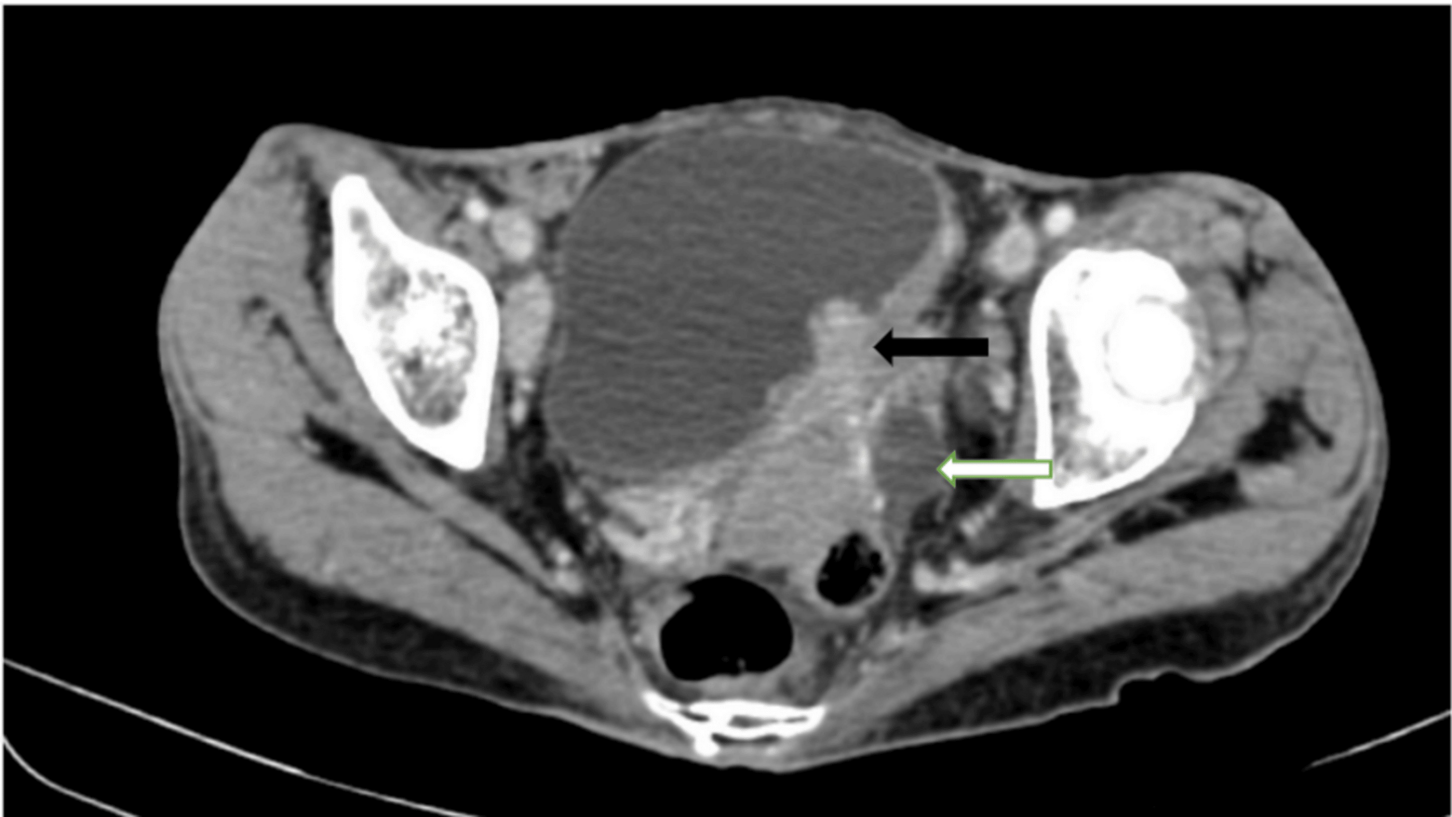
Contrast-enhanced computed tomography axial section shows heterogeneously enhancing polypoidal soft tissue lesion (black arrow) arising from the left lateral wall of the urinary bladder involving the left vesicoureteric junction causing upstream dilatation of the left ureter (white arrow)
